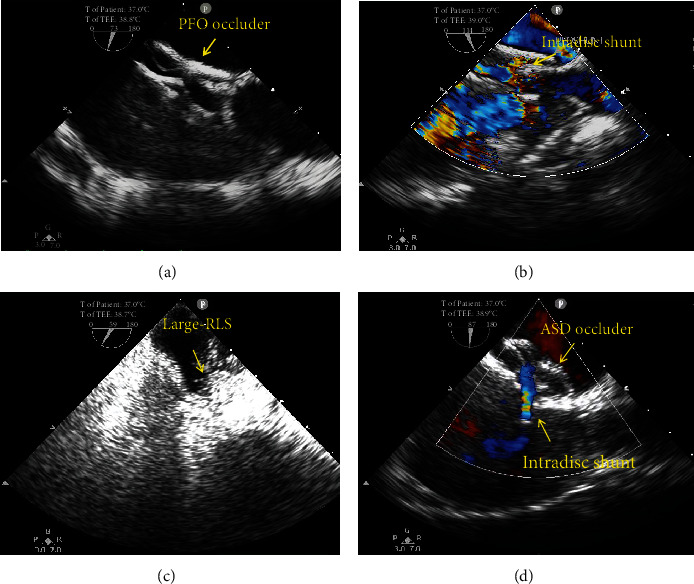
Two patients with a large residual RLS underwent TEE and c-TEE at 12 months after the procedure. (a) An oversized PFO occluder device. (b) An intradisc residual shunt. (c) A large residual RLS from the middle of the PFO occluder device. (d) An oversized ASD occluder device and an intradisc residual shunt. c-TEE: contrast transesophageal echocardiography; PFO: patent foramen ovale; RLS: right-to-left shunt; TEE, transesophageal echocardiography.
